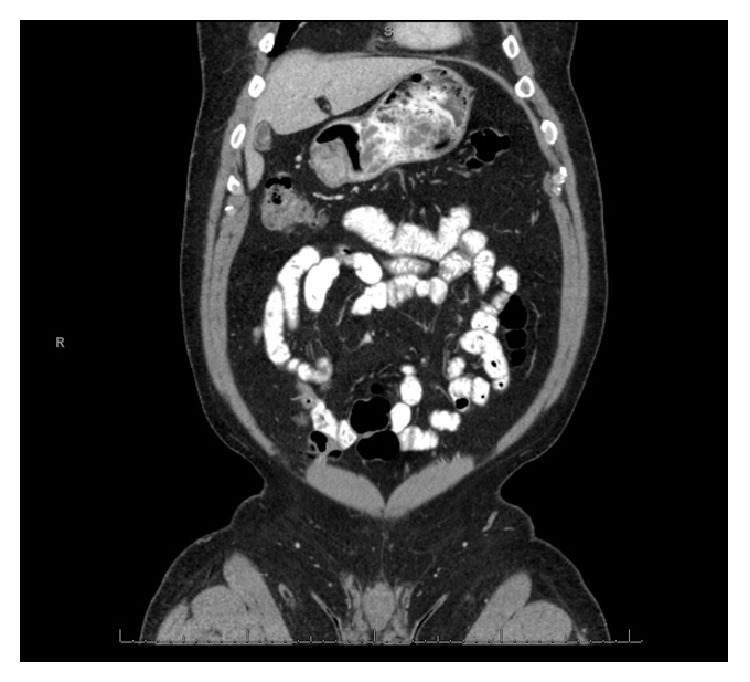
Image from a computed tomography scan demonstrating an endophytic tumor arising in a challenging location in the gastric antrum.
